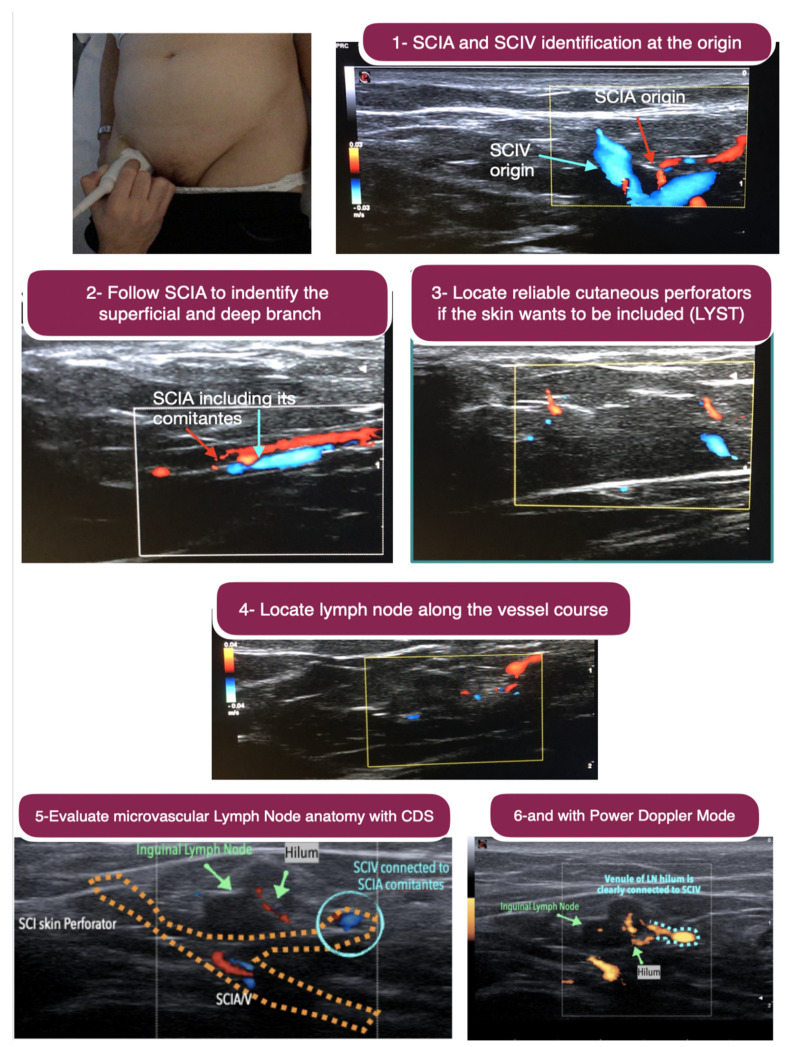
Step-by-step ultrasound preoperative planning of inguinal vascularised lymph node transfer. Above, left: The 18-MHz linear probe is used. Above, right: Step 1. Identification of the superficial circumflex iliac artery and its comitantes veins (SCIA) as well as superficial circumflex iliac vein (SCIV) at their origin (i.e., femoral artery and vein). Second row, left: Step 2. The SCIA is followed to identify the superficial and deep branch. Second row, right: Step 3. Location of reliable cutaneous perforator from the selected branch (superficial or deep) if the skin wants to be included in the flap. Third row: Step 4. Locate lymph nodes along the vessel course. The superficial branch is usually the branch with most nearby lymph node. Below, left: Step 5. Once the lymph node is identified, the microvascular anatomy is evaluated using the colour-duplex mode to understand the lymph node arterial input and venule output. In some cases, the venule output belongs to SCIV rather than SCIA comitantes, and this information is very valuable in flap planning and execution. Below, right: When the colour-duplex mode is not very clear, the power doppler mode can be employed for clearer evaluation. Comparing the last two images, it is clear that the power doppler is more powerful in seeing that the venule output of the lymph node is clearly connected to SCIV.
